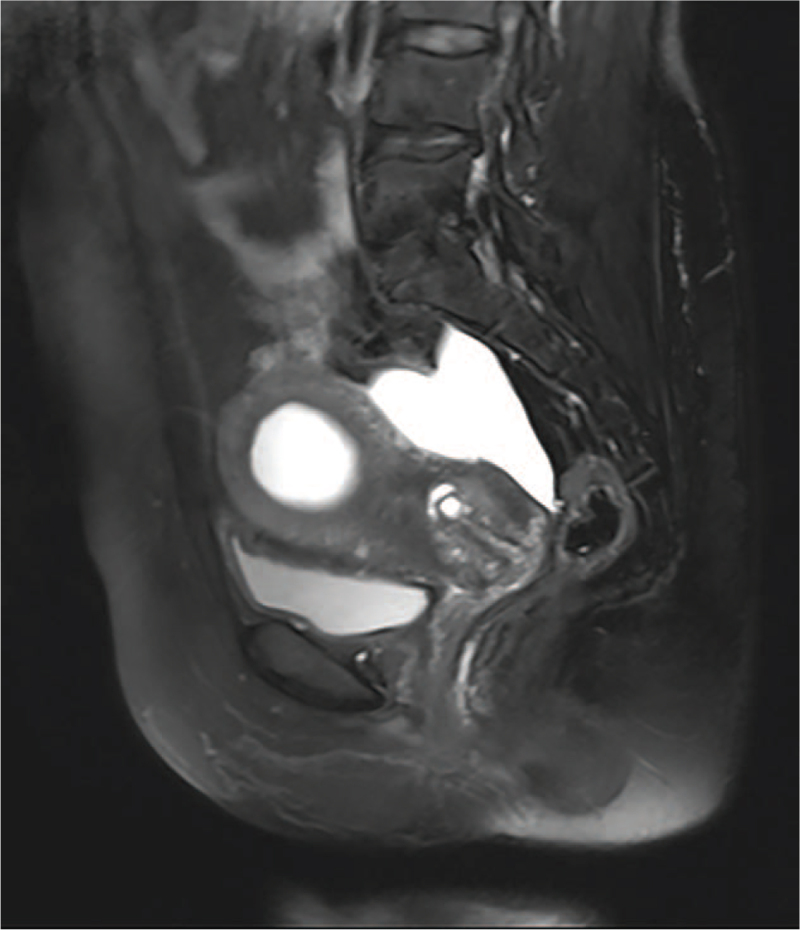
Thickening of the posterior wall of the cervix with a mass-like signal shadow suggested by MRI. MRI = magnetic resonance imaging.
